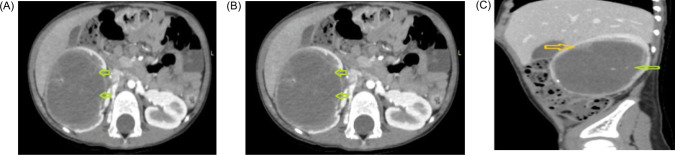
Contrast-enhanced CT scan (abdomen and pelvis). (A) Axial noncontrast images, showing a heterodense solid cystic mass in the right kidney, showing the presence of calcifications (yellow arrow). (B) and (C) Axial and sagittal CECT images showing a cystic lesion in the right kidney with thin enhancing internal septations (green arrow) and peripherally placed solid components (yellow arrow).
